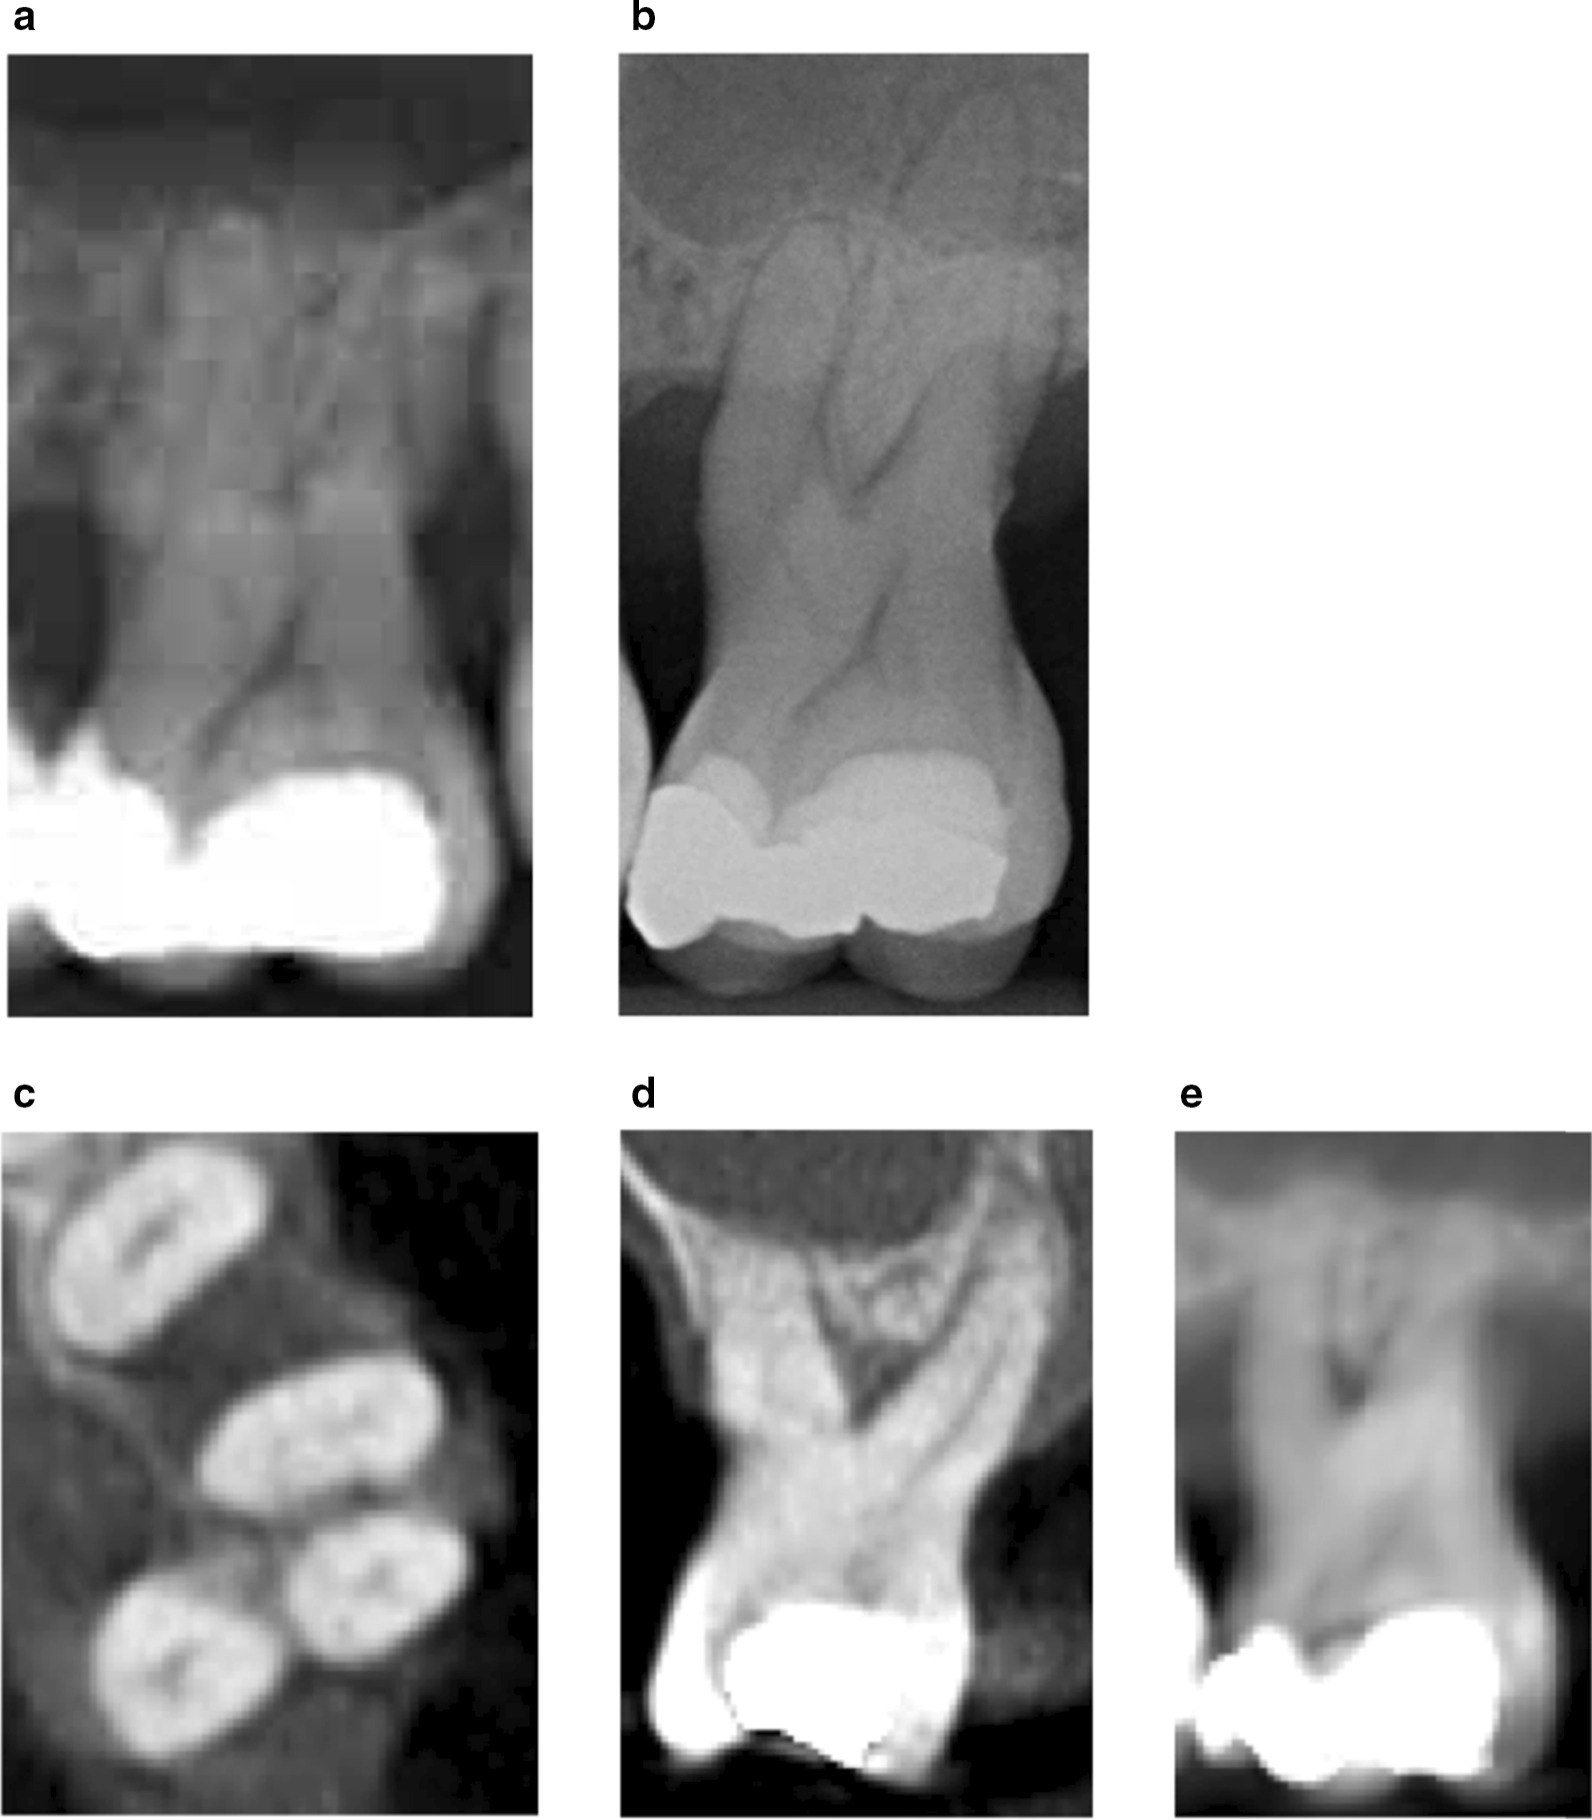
Clinical example of a maxillary molar with FI Grade III at the mesiopalatal site. a Enlarged panoramic radiograph, b peri-apical radiograph, c CBCT: axial slide, d CBCT: coronal slide, e CBCT: sagittal slide
